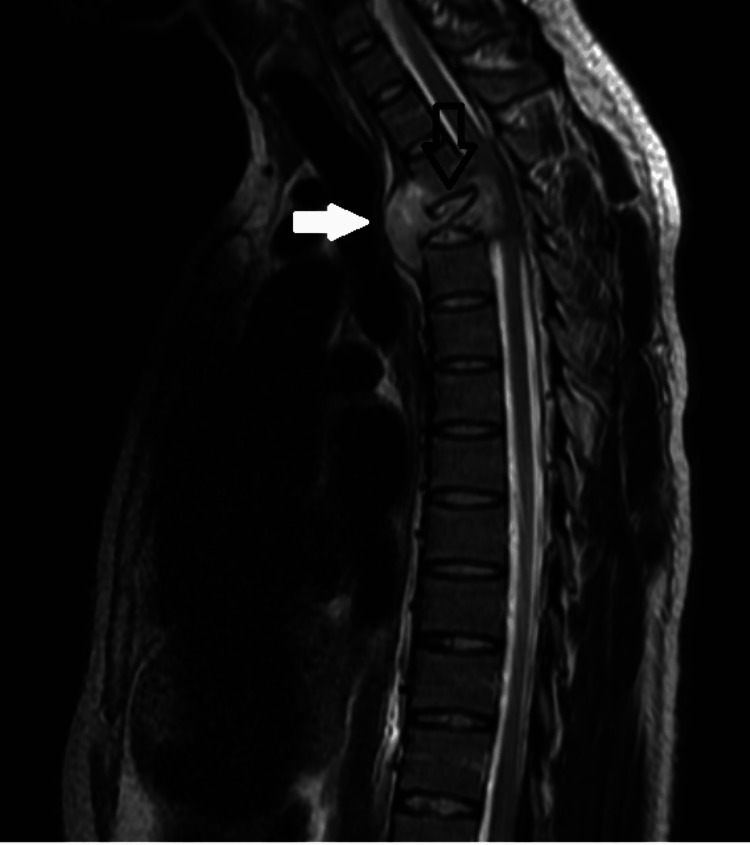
MRI showing the metastatic compression fracture (black arrow) and a prevertebral paraspinous soft tissue mass (white arrow) MRI: magnetic resonance imaging.
